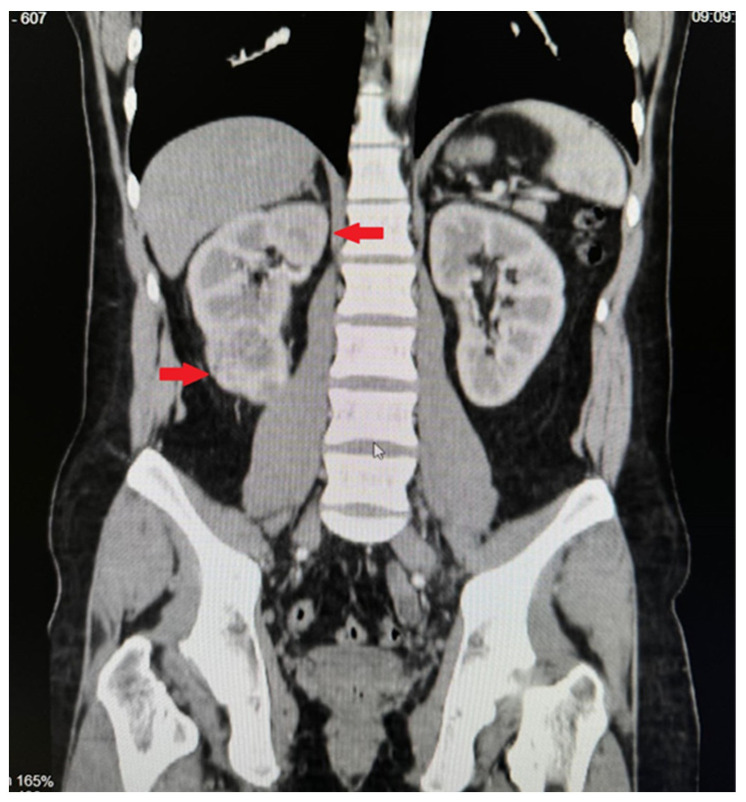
CT image. Tumour mass in the inferior part of the right kidney.
